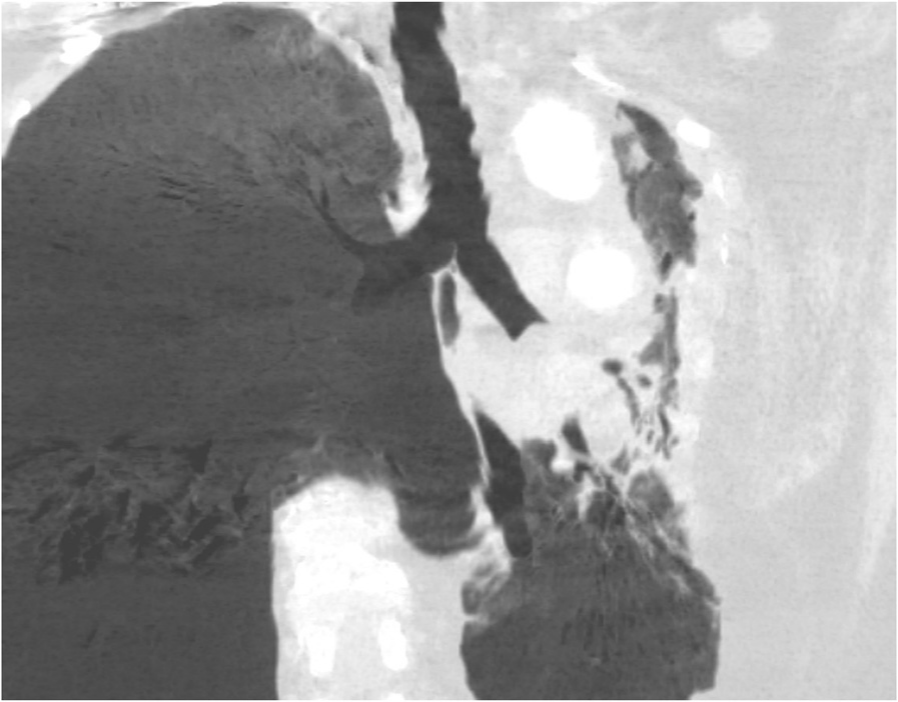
2 D chest CT image of the intrabronchial mass located 3 cm from the main carina.
